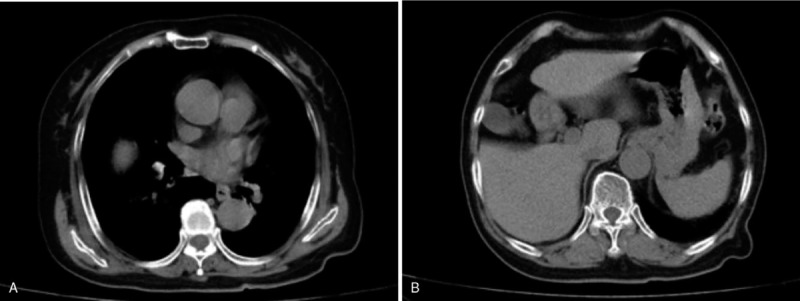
Preoperative CT shows no abnormality of diaphragm or colon (A and B).
